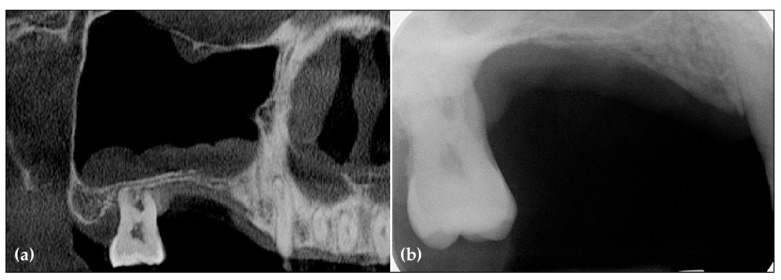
(a) The panoramic image and CBCT scans revealed severe vertical bone resorption along with a hopeless third molar. Additionally, a retention cyst was observed in the maxillary sinus; (b) The preoperative periapical radiograph revealed severe vertical ridge deficiency.
